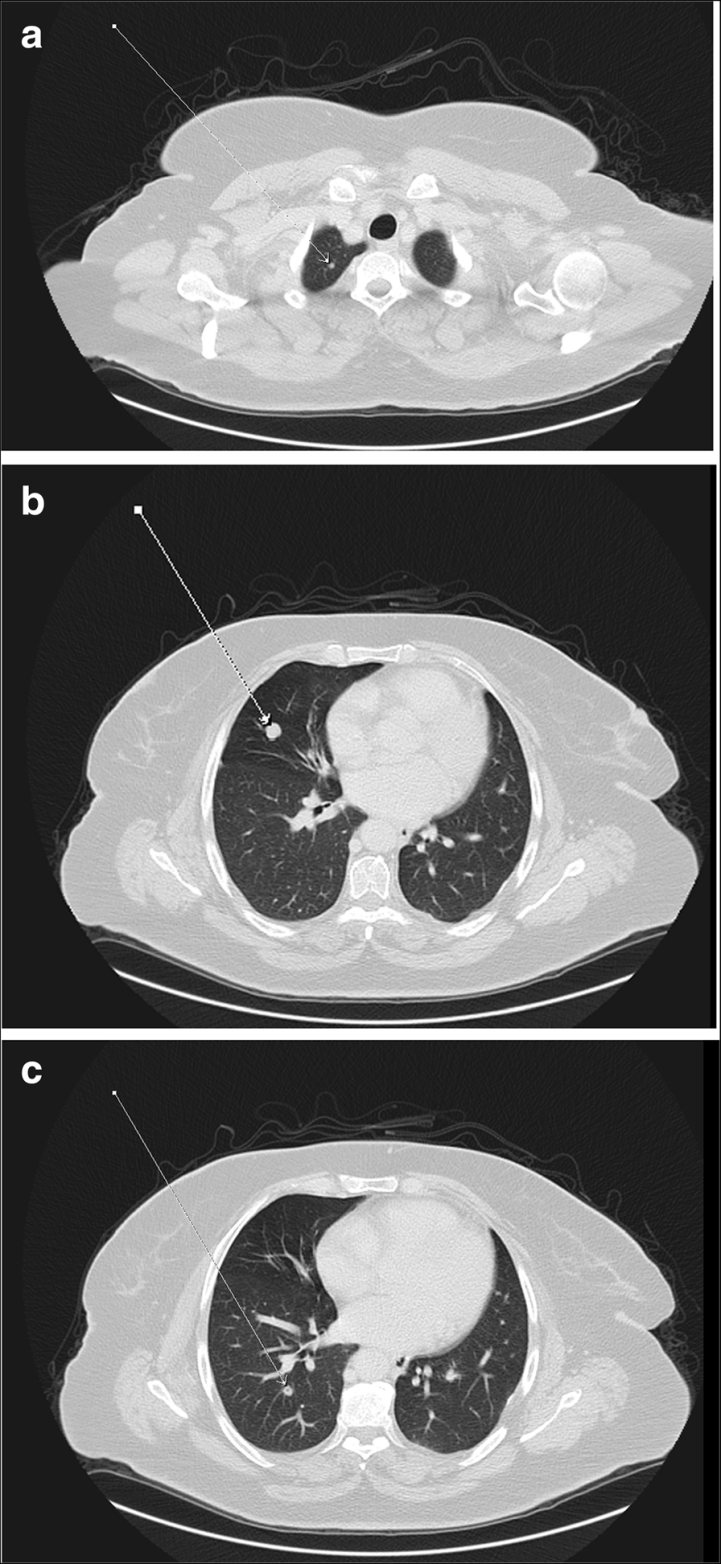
Pre-operative chest CT scan showing the location of three pulmonary nodules in patient 1. Pre-operative chest CT scan – axial view – pulmonary window, showing three right pulmonary nodules located on a the superior lobe, b lateral part of the middle lobe, and c inferior lobe. All indicated by white arrow
